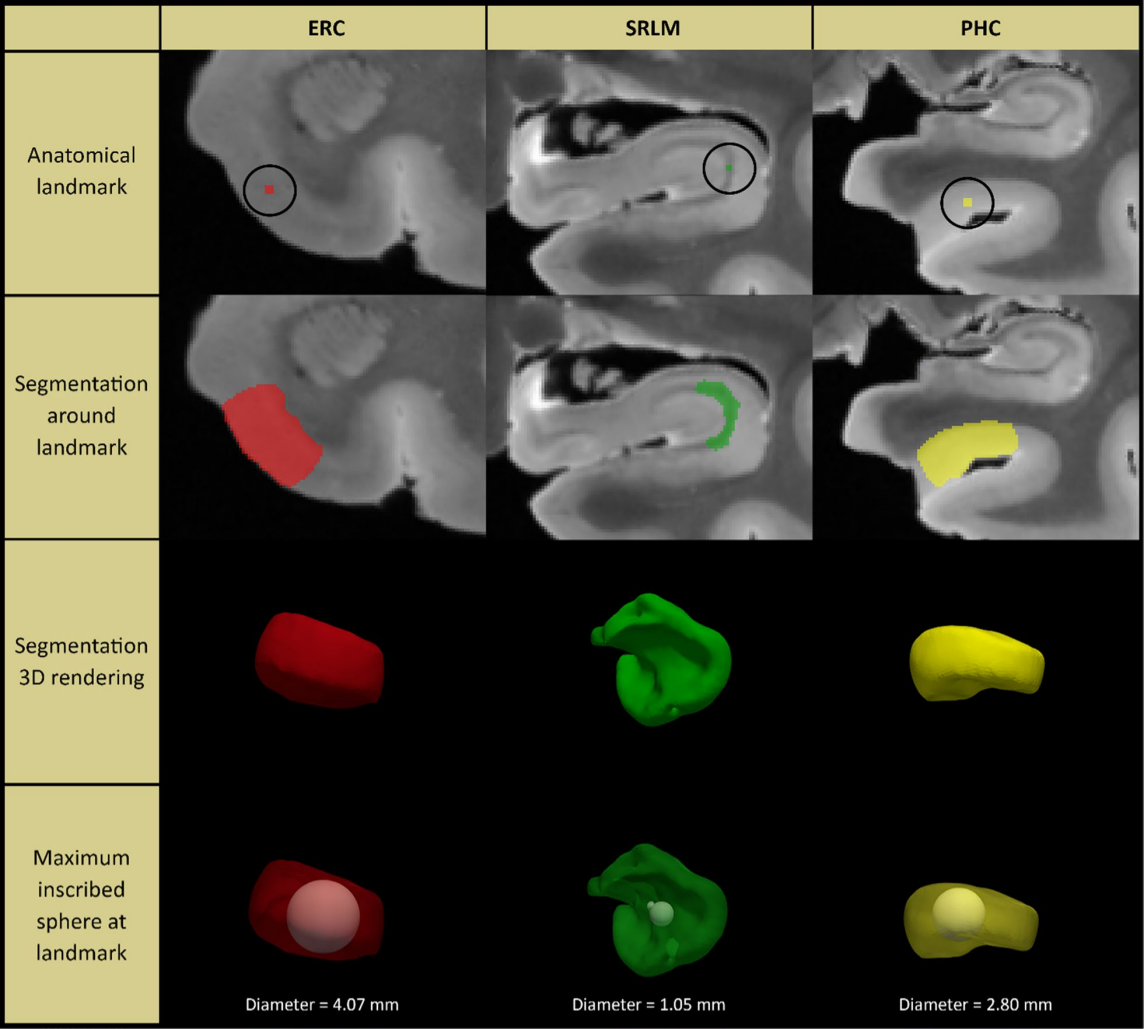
Method for obtaining thickness measures from high-resolution post-mortem MRI in medial temporal lobe subregions. For each anatomical location (indicated by a dot; first row), cortical thickness was measured by using semi-automated active contour segmentation in ITK-SNAP (with possible manual correction) to segment a portion of the surrounding gray matter extending ~ 5–10 mm from the dot in all directions (second and third row). Thickness at each location was measured as the diameter of the sphere overlapping the dot, fully contained in the gray matter segmentation, and having maximum possible radius (fourth row). Segmentations and fitted spheres were visually inspected for quality assurance. See supplementary Methods for details. Note that we only show three examples in this figure. For this study we performed thickness measurements for seven subregions and averaged thickness measurements over two locations for each subregion, see Additional file 1: Fig. 1. ERC = entorhinal cortex; SRLM = stratum radiatum lacunosum moleculare; PHC = parahippocampal cortex
